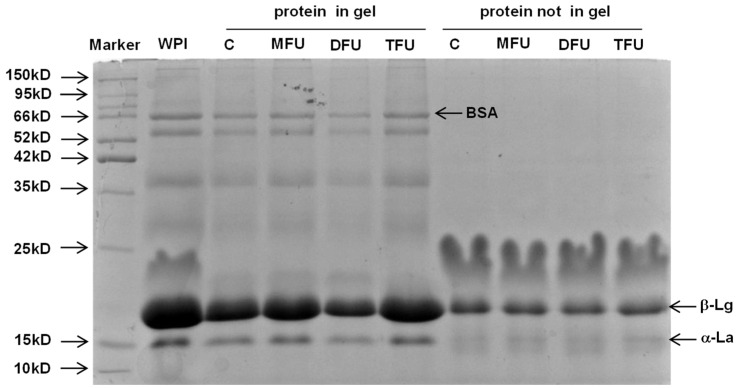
SDS-PAGE of protein distribution in whey protein gels with different ultrasound pretreatment (C: control without ultrasound, MFU: mono-frequency ultrasound, DFU: dual-frequency ultrasound, TFU: tri-frequency ultrasound).
